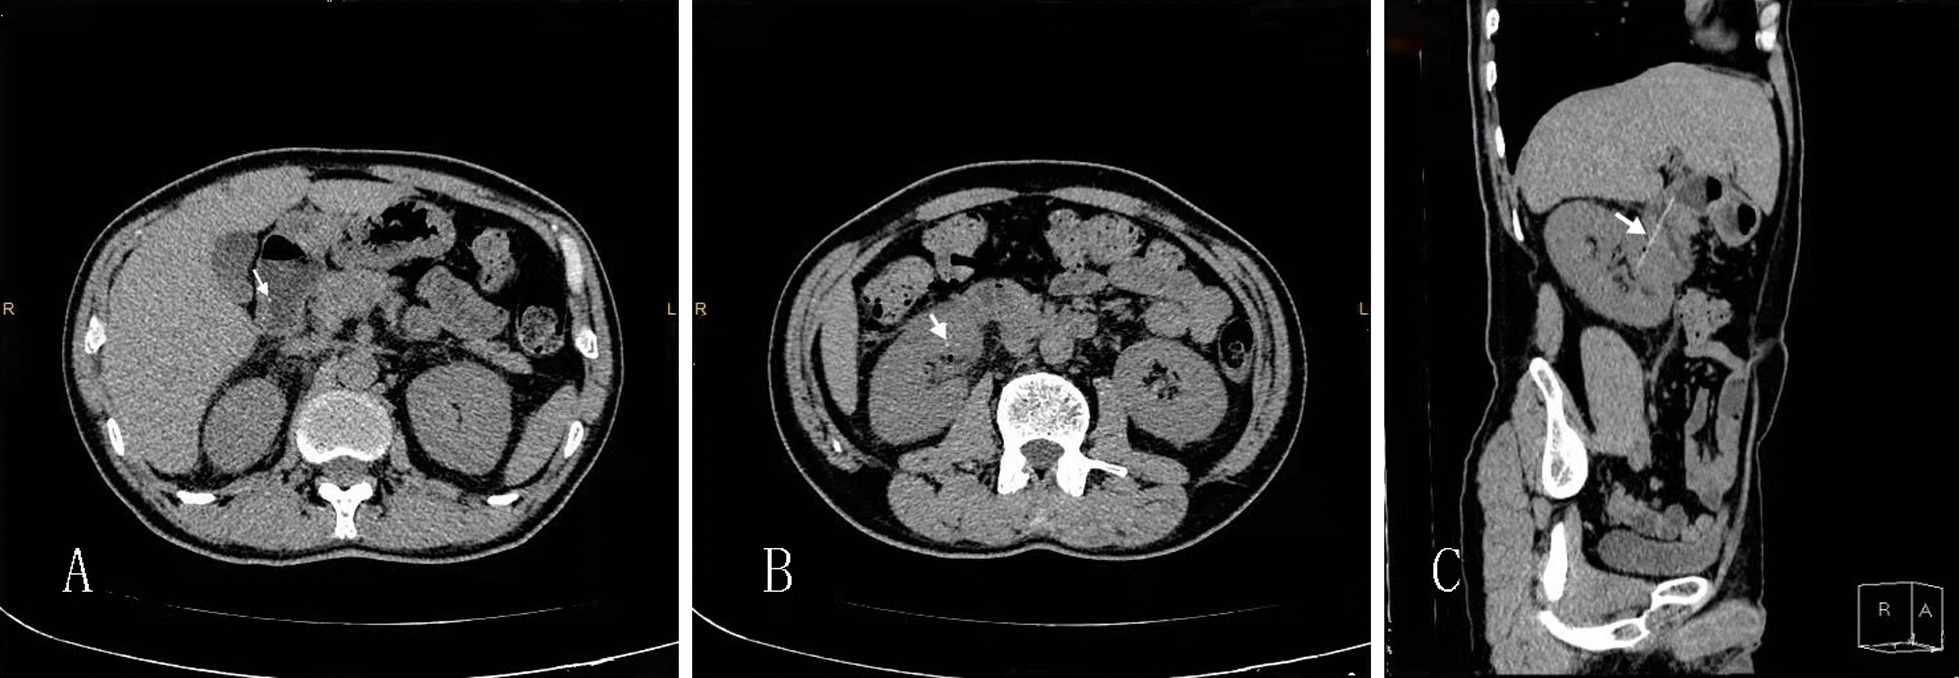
The two ends of toothpick are respectively showed as high density dots in duodenum (A: arrow) and the right kidney (B: arrow). The toothpick shown as linear high density is penetrated into the right kidney in the CT after 3D reconstruction (C: arrow). The “R” and “L” in pictures A and B represent the right and left respectively. In picture C, “R” and “A” represent the right side and ventral side respectively
